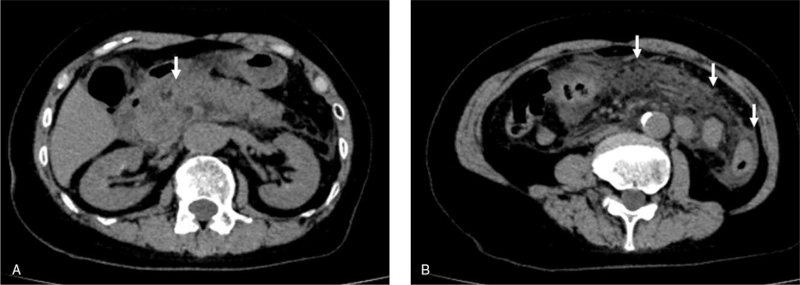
(A) Computed tomography (CT) scan demonstrating diffuse enlargement of the pancreas and ill-defined parenchymal contours (arrow). (B) CT showed fat stranding extending to below the kidneys bilaterally (arrow).
